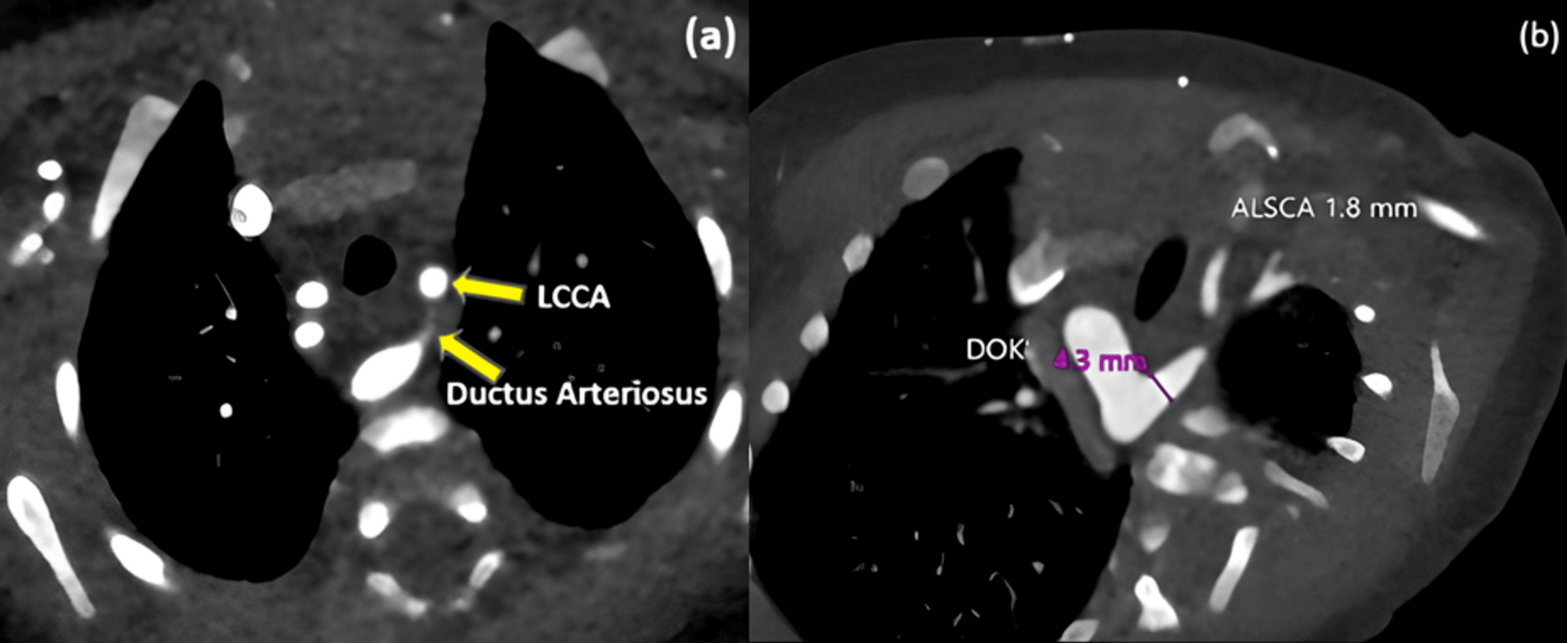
Contrast-enhanced CT depicting vascular anatomy (a) Axial Image showing LCCA with a probable origin from the ductus arteriosus. (b) Reconstructed image showing the right aortic arch, DOK, and an aberrant left subclavian artery. This configuration is consistent with a complete vascular ring. ALSCA: Aberrant left subclavian artery; DOK: Diverticulum of Kommerell; LCCA: Left common carotid artery
